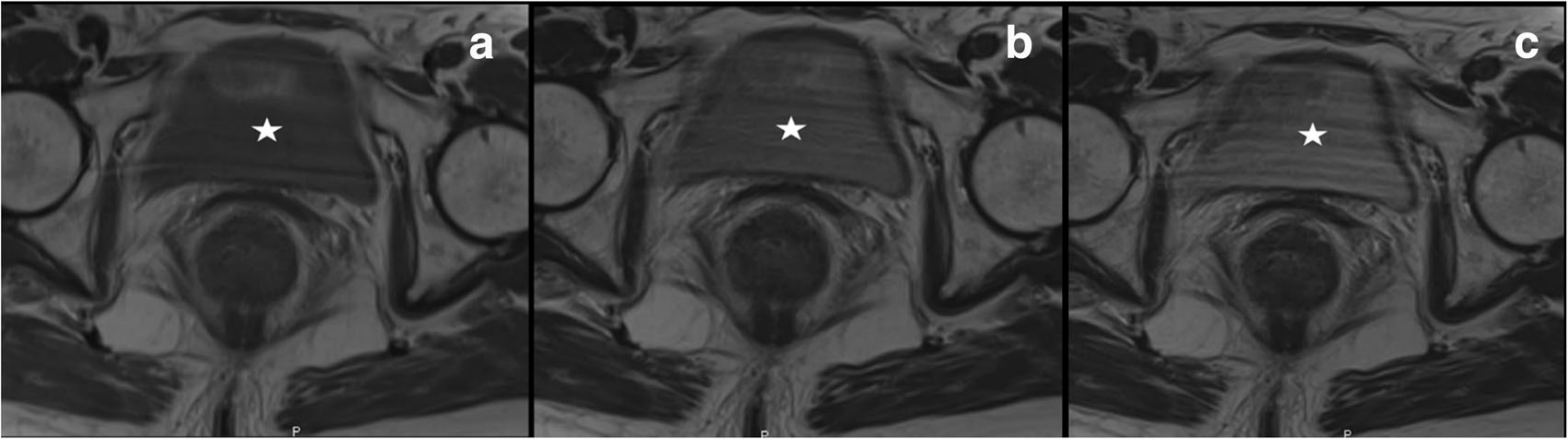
Axial T2-weighted images of the pelvis, acquired keeping unchanged all the parameters, with only exception of the echo time, which was 34 ms in (a), 90 ms in (b), and 134 ms in (c), showing that even one single parameter can change the signal intensity of tissues and fluids, as clearly depicted by the signal of the bladder (white star), with higher and higher signal intensity from a to b to c
